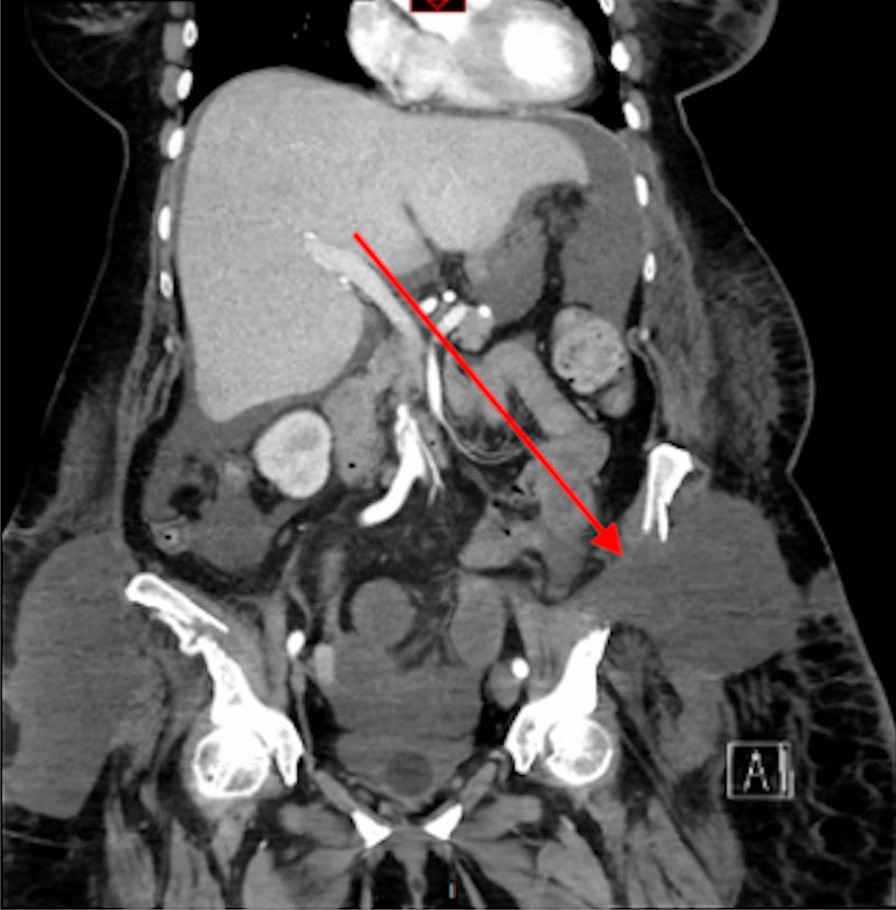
Abdominal CT scan: interfragmentary hernia (arrow)
